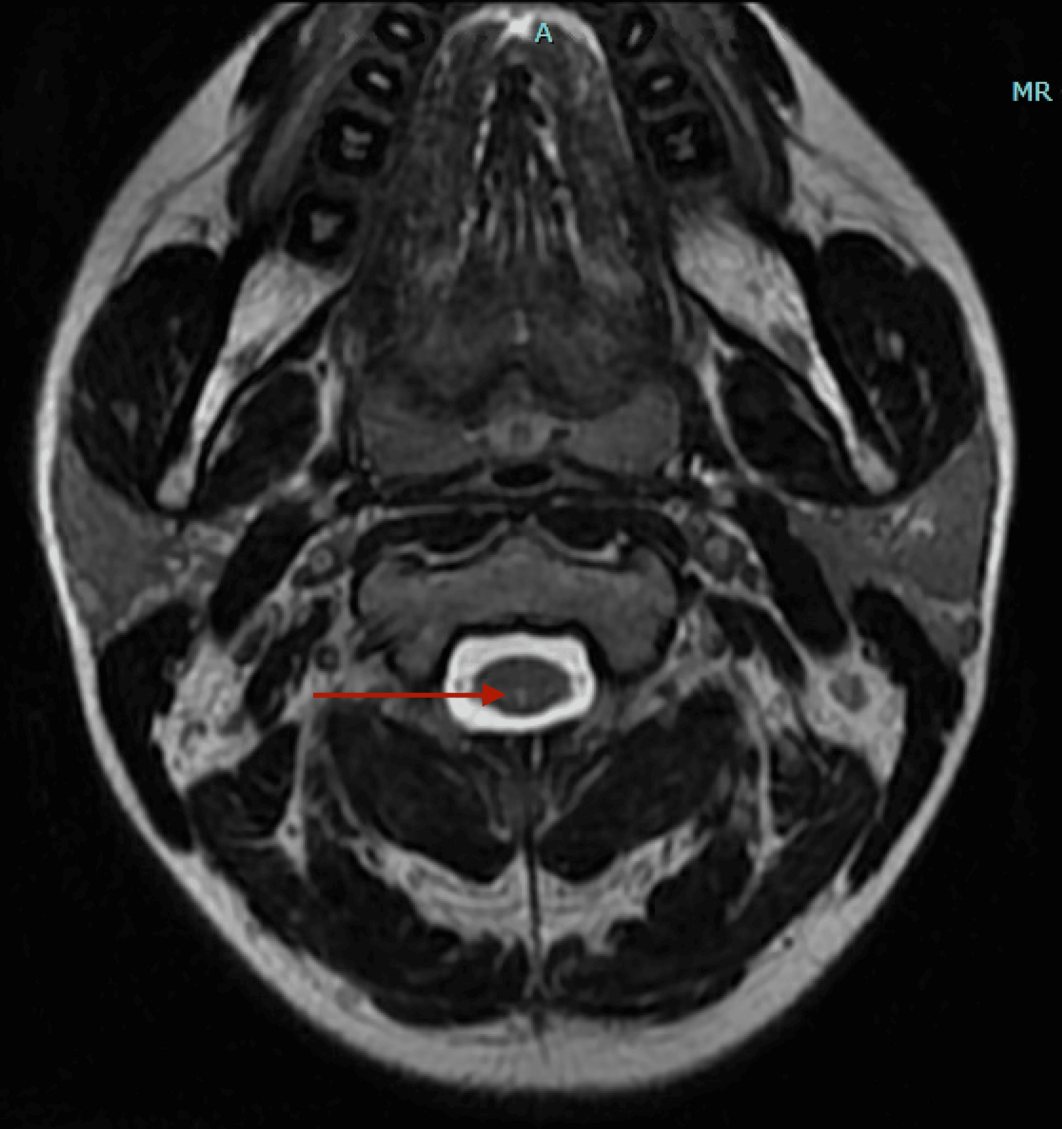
Dedicated MRI of the C-spine demonstrating small subtle short-segment T2 hyperintense signal affecting the dorsal columns C2-C4 concerning for subacute combined degeneration of the cord MRI: magnetic resonance imaging
